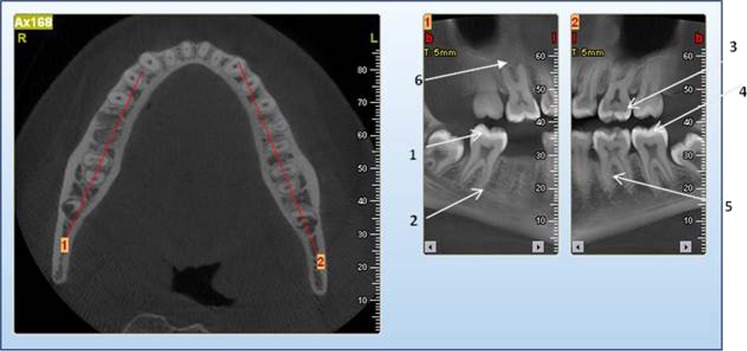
Cropped CBCT image of Individual CPT 1 with features of mild DI which include hypotaurodontism (1), thin short roots of molars (2), cervical constriction of crowns of molars (3), abnormal occlusal anatomy (4), and focal areas of narrowed and occluded root canals (5). Periapical radiolucencies involving teeth 17 and 27 (6)A compound heterozygous mutation, c. [831delC]; [831dupC] was identified in three siblings. This is a frameshift DNA variant in exon 5 of *FKBP10* which is predicted to alter the protein sequence by substituting a Glycine residue with an Alanine at position 278 of the 65 kDa FK506 Binding protein 10. A premature termination codon results in the loss of 286 amino acid residues.An intraoral examination of these affected persons revealed no clinically apparent features of DI in their primary and secondary teeth. Due to the lack of radiological facilities, the presence or absence of DI could not be confirmed or negated (Figs. 3 and 4).
